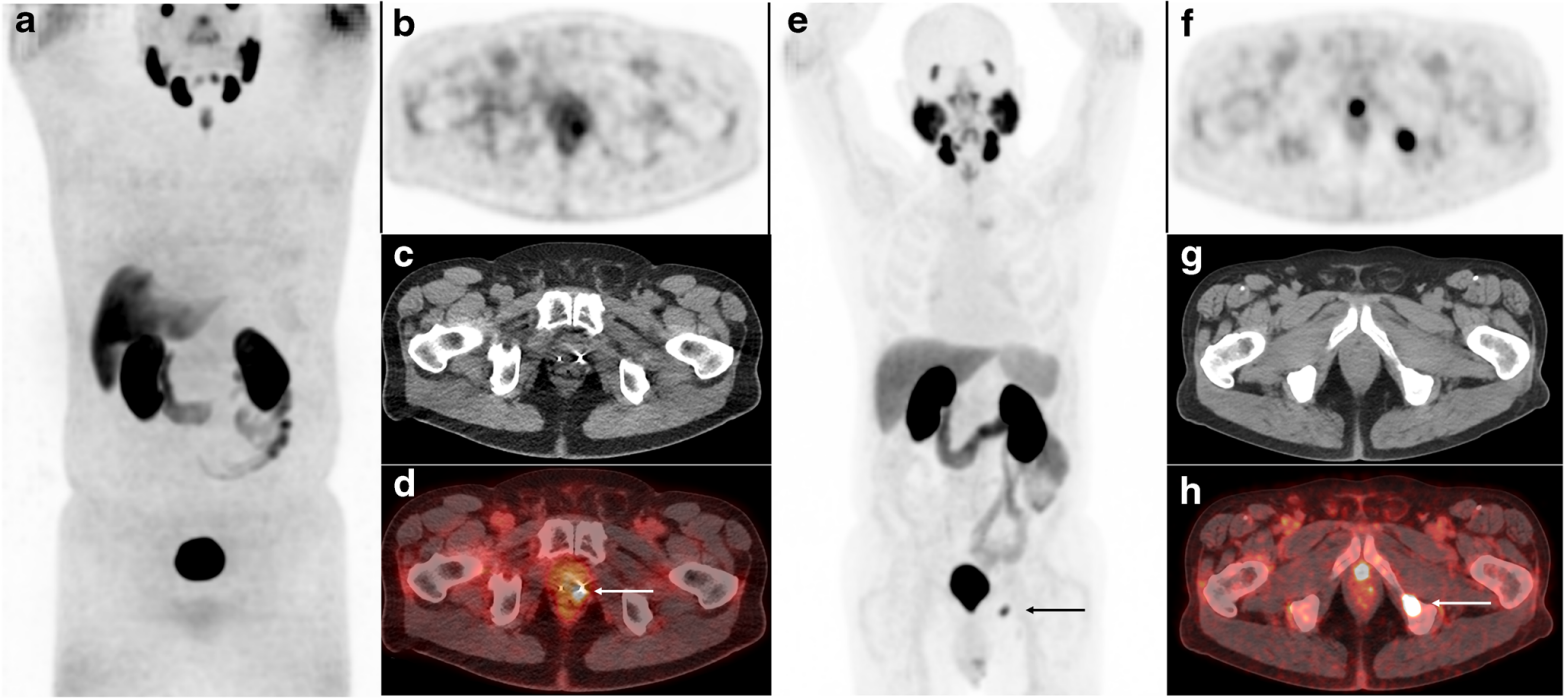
PSMA PET/CT images (maximum intensity projection (MIP), left panel; PET, upper panel; CT, mid-panel; fused PET/CT, lower panel) in 2 patients with BCR. a–d Patient 1: A 67-year-old patient, with a rising PSA of 1.1 ng/mL, 5 years after EBRT (PSA-nadir 0.1 ng/mL). (Delayed) step-up (systemic) hormonal therapy was chosen as the preferred treatment. However, the performed PSMA PET/CT showed local recurrent disease (miTr), resulting in a change of management from systemic treatment to local treatment. e–h Patient 2: A 76-year-old patient, with a rising PSA of 0.8 ng/mL, 2 years after RARP (PSA nadir <0.1 ng/mL). Salvage radiation therapy to the prostatic fossa (local treatment) was chosen as the preferred treatment. However, the performed PSMA PET/CT showed one bone metastasis (miM1b) in the left os ischium, resulting in a change of management from local treatment to metastasis-directed therapy
